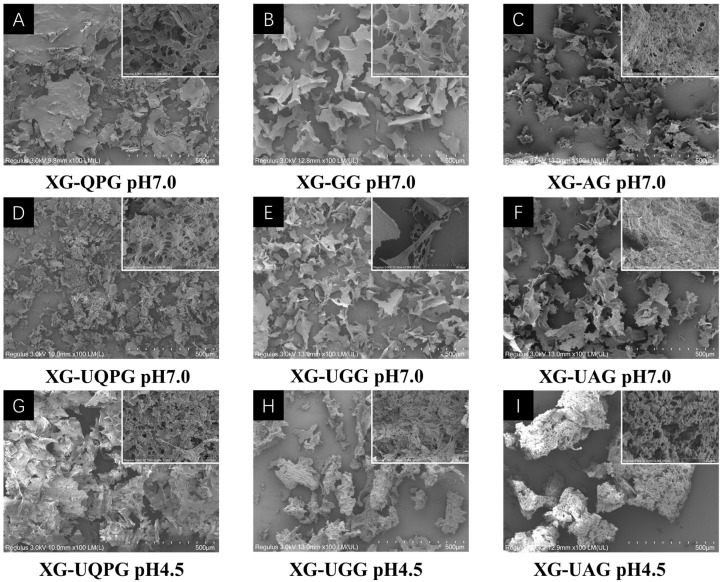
Effects of ultrasound and protein components on the microstructure of XG-QPG pH 7.0 (A), XG-GG pH 7.0 (B), XG-AG pH 7.0 (C), XG-UQPG pH 7.0 (D), XG-UGG pH 7.0 (E), XG-UAG pH 7.0 (F), XG-UQPG pH 4.5 (G), XG-UGG pH 4.5 (H), XG-UAG pH 4.5 (I).
